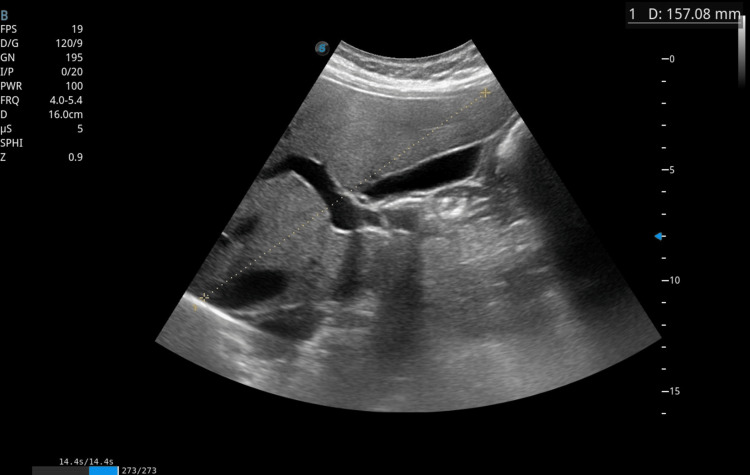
Abdominal ultrasound showing splenomegaly (approximately 15.7 cm) in a patient with compound heterozygous sickle cell-beta thalassemia and chronic hemolytic anemia.
